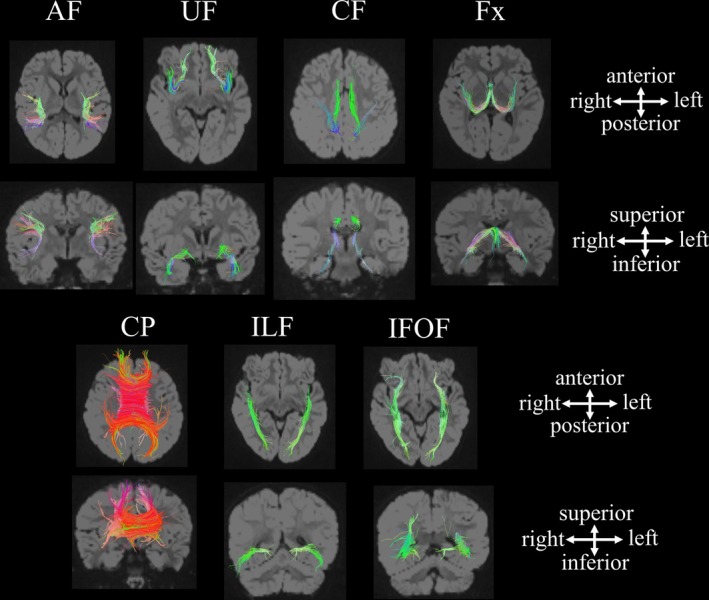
Ventral and front views of high‐angular resolution diffusion MR imaging tractography of a 2.5‐year‐old neurotypical girl (reproduced with permission to reuse [102]). AF, arcuate fasciculus; CF, cingulum fasciculus; CP, callosal pathway; Fx, fornix; IFOF, inferior fronto‐occipital fasciculus; ILF, inferior longitudinal fasciculus; UF, uncinate fasciculus.
